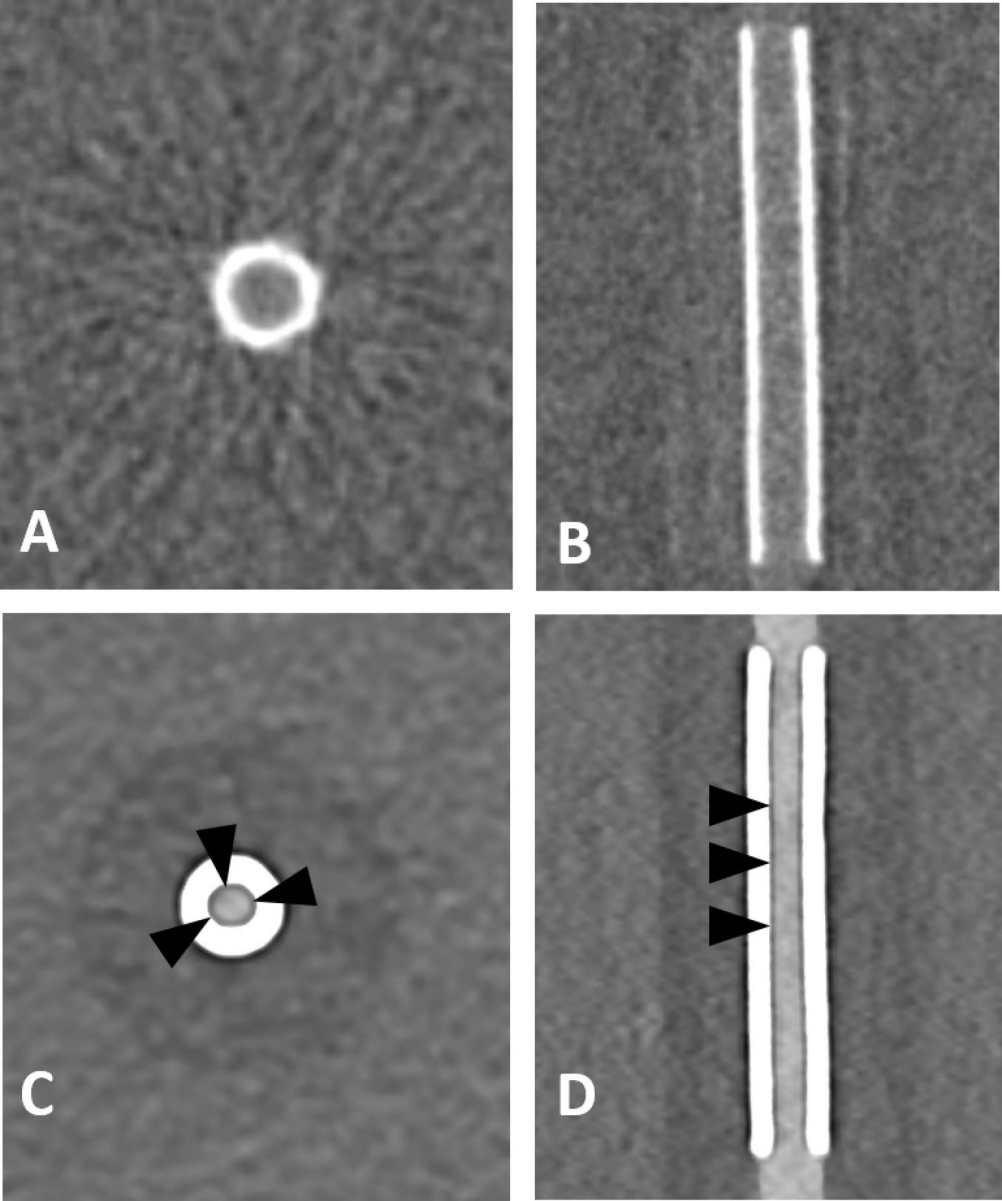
Flat panel CT (A, B) and photon-counting detector CT (CTDIvol 20 mGy; C, D) with axial and sagittal reconstructions of a Silk Vista 2.5 × 15 mm flow diverter. Compared to FP-CT, PCD-CT shows a smaller apparent lumen and a hypodense artifact adjacent to the stent wall (arrowheads). Window settings were manually optimized for contrast: A, B) W: 700 HU, L: 2000 HU; C, D) W: 250 HU, L: 700 HU.
